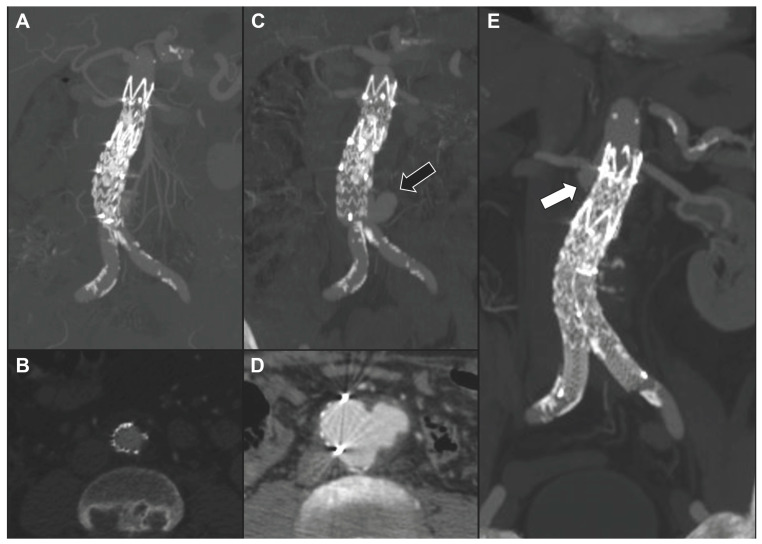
One-month computed tomography angiography (CTA) showing a good result after straight EVAR (with 2 cuffs) for contained ruptured infrarenal atheromatous plaque in maximum intensity projection (A) and axial view (B). The same patient experienced, after 5 months, distal disease progression with rupture of the aortic bifurcation (black arrow) (C,D). The 55-month CTA showed the exclusion of the infrarenal lesion with initial degeneration of the proximal aortic neck (white arrow) (E).
